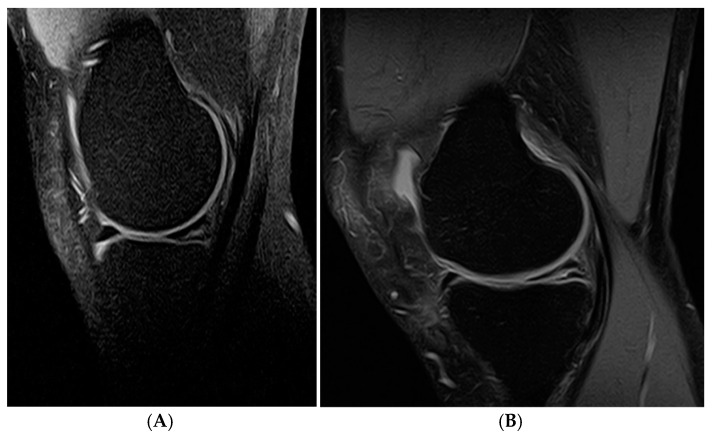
(A,B) MRI images of menisci lesions.
